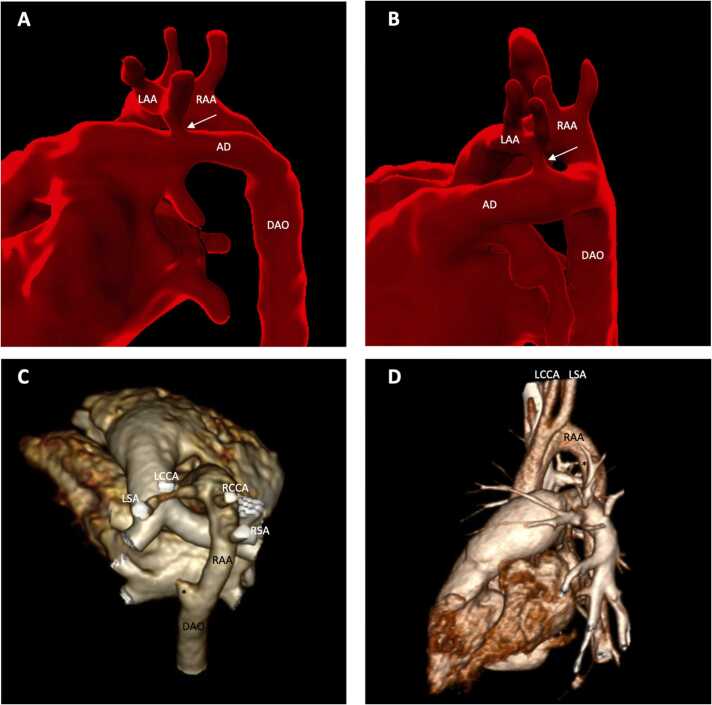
DAA with LAA isthmal atresia—CT suggestive of RAA. Visualization of LAA isthmus can be limited on 2D fetal echocardiography when there is a complex spatial relationship or superior-inferior pathway and insertion of the aortic isthmus into arterial duct and severe displacement of the isthmus (arrow A and B). On postnatal CT, the diagnosis appears to be of a single RAA with mirror image branching for both patients (A/C and B/D). The postero-inferior course of the LAA and four-vessel sign are not present (C and D). The clue to the presence of an atretic LAA is the diverticulum on the DAO (*). Both infants were symptomatic and had moderate to severe airway compression diagnosed on bronchoscopy. In both, surgery confirmed the presence of an atretic LAA in addition to the presence of a ligamentum arteriosum. *AD* arterial duct, *DAO* descending aorta, *LAA* left aortic arch, *LCCA* left common carotid artery, *LSA* left subclavian artery, *RAA* right aortic arch, *RCCA* right common carotid artery, *RSA* right subclavian artery, *DAA* double aortic arch, *CT* computed tomography, *2D* two-dimensional.
